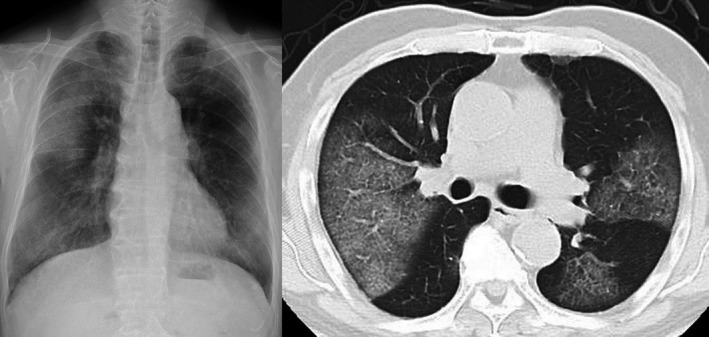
Chest X‐ray and computed tomography images showed bilateral ground‐glass opacities
